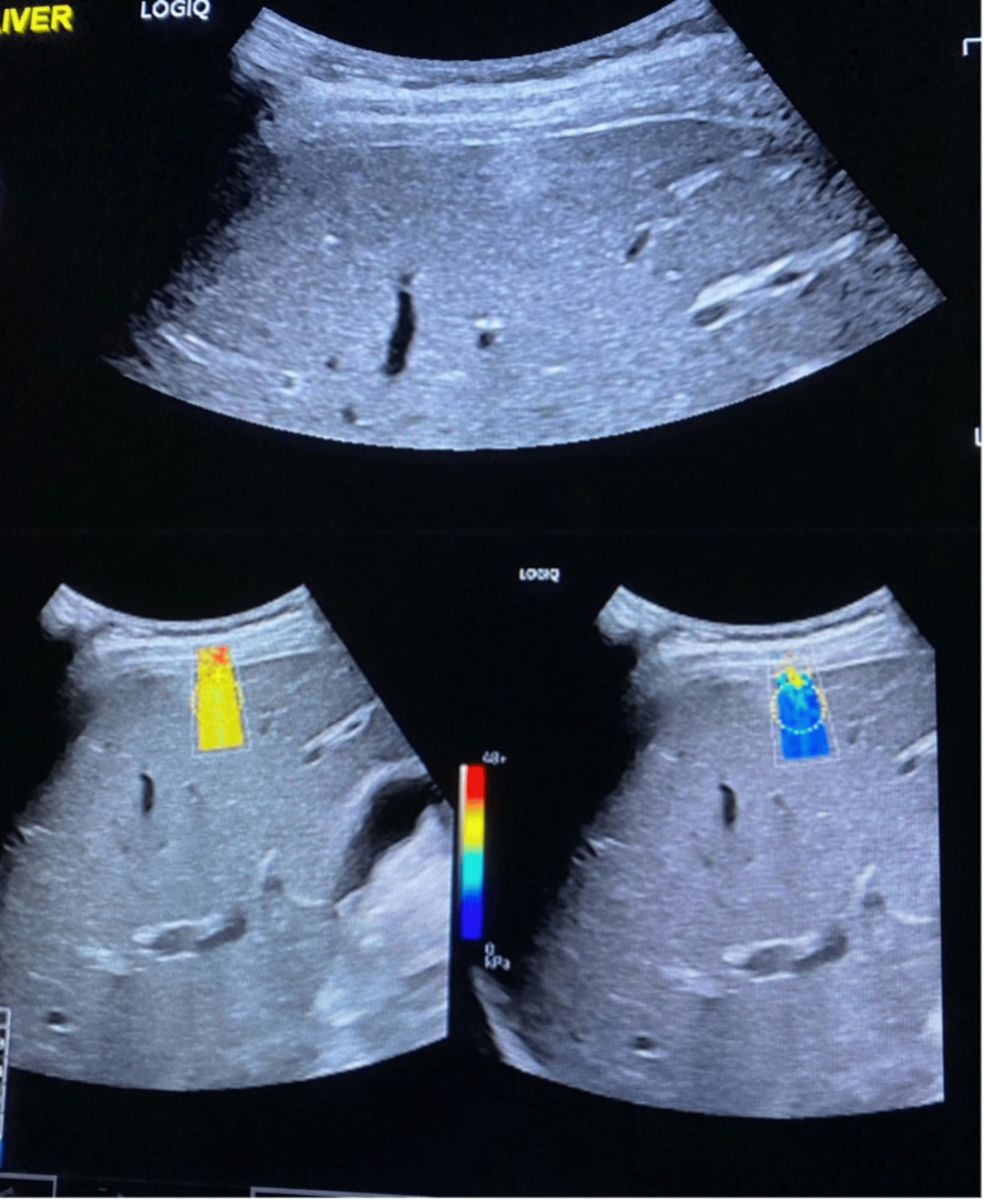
2D-SWE in non-malignant lesion (liver haemangioma) in the liver parenchyma (lesion stiffness 3.47 kPa) The ultrasound images shown are part of our team's original work and were obtained by the authors as part of this study.
